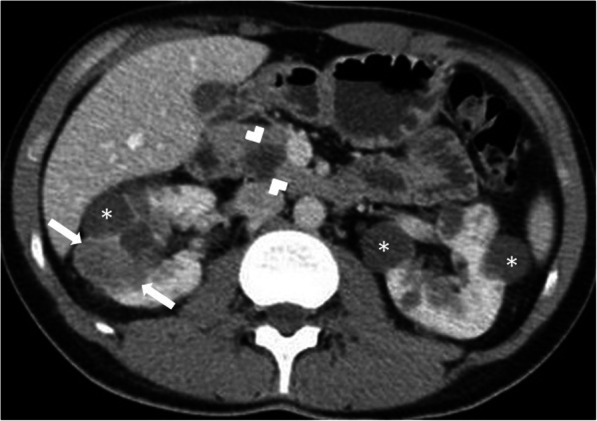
A 43-year-old male patient with known VHL underwent a follow-up CT exam. Axial plane post-contrast CT image shows several renal cysts (asterisks) in both kidneys. Also note is made of a new focus of clear cell RCC (surgically confirmed) in the right kidney (arrow). A pancreatic cyst in the uncinate process was also detected (arrowheads)
